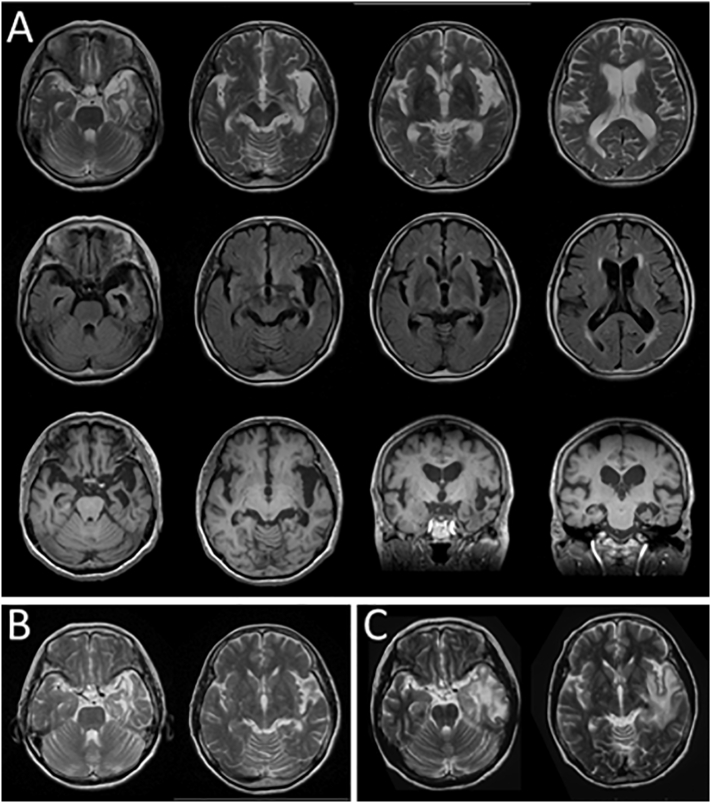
Magnetic resonance imaging data. (A) Axial T2-weighted imaging (T2WI), axial fluid-attenuated inversion-recovery images, and axial and coronal T1-weighted imaging revealed the previously diagnosed venous thrombosis in the left anterior temporal lobe. (B) The findings of T2WI were almost unchanged from those imaged 7 years before admission. (C) The T2WI images taken from when she was 60 years old showed a high-intensity area in the left temporal lobe and insula, indicating venous thrombosis with brain edema.
